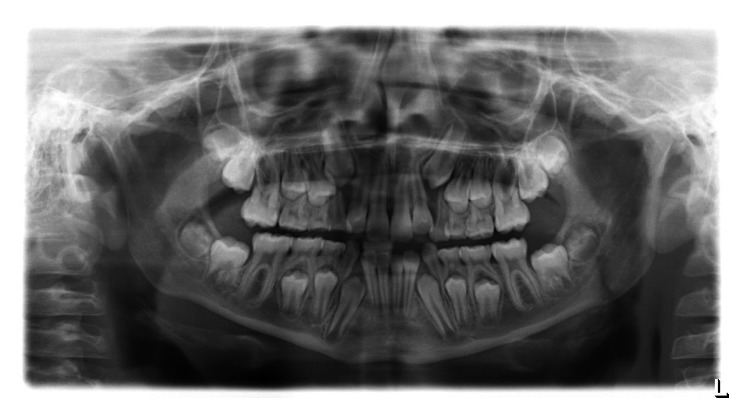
OPG radiograph taken 4 years previously with no evidence of the calcified mass seen on the later radiographs.
